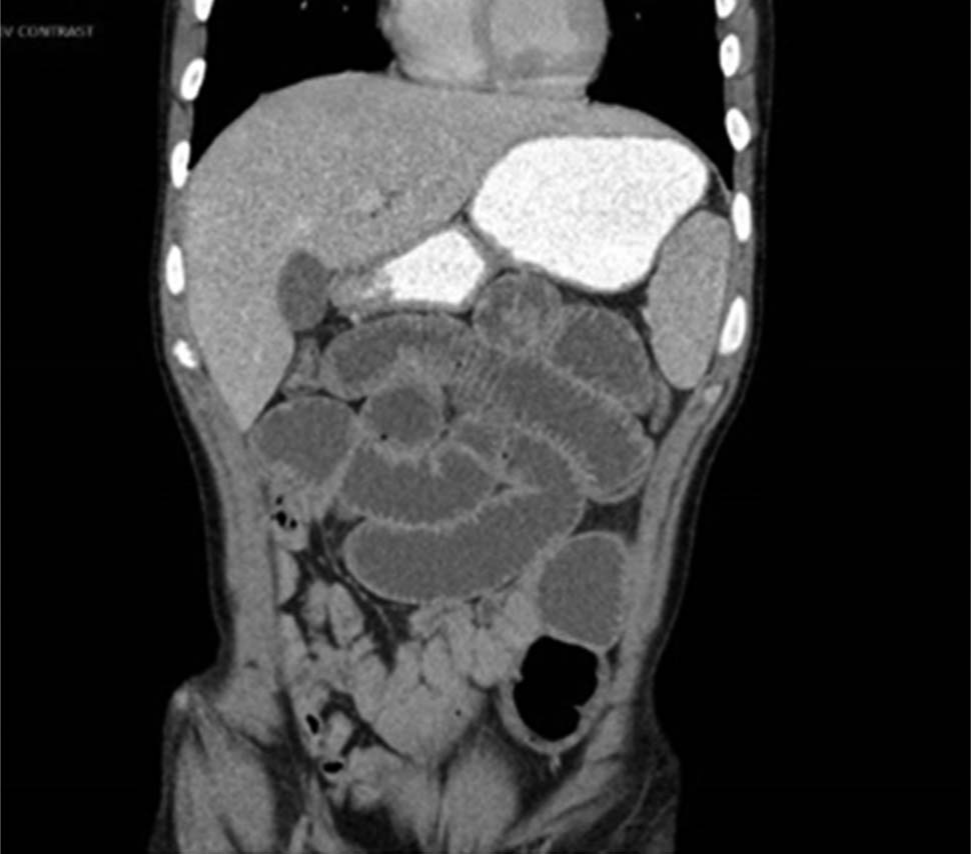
Coronal computed tomography scan showing an acute noninflammatory small bowel obstruction.
